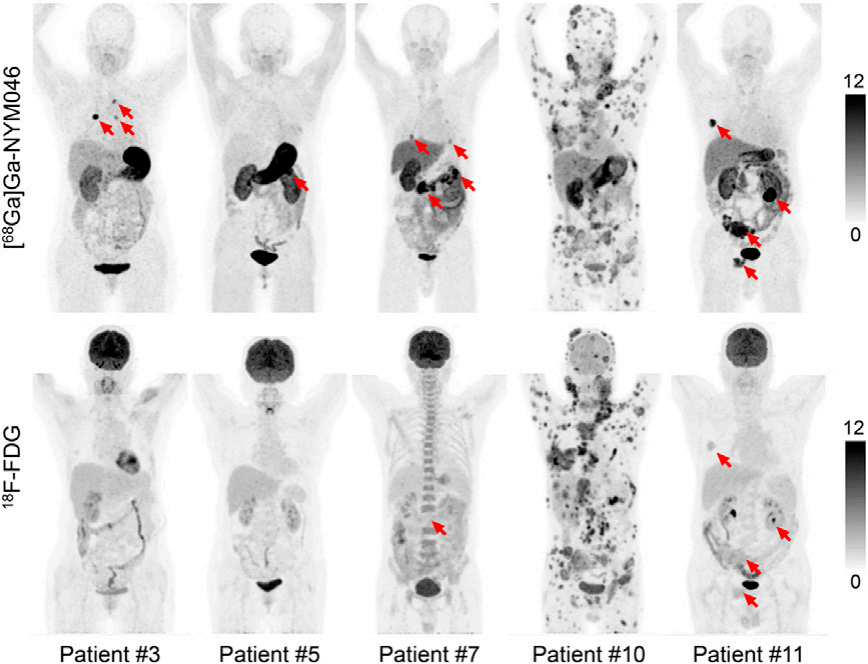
Representative maximum-intensity projections of 5 patients (patients 3, 5, 7, 10, and 11) comparing [^68^Ga]Ga-NYM046 and ^18^F-FDG. Both tracers showed specific retention in bone and lymph node metastases (patients 10 and 11). [^68^Ga]Ga-NYM046 PET/CT outperformed ^18^F-FDG PET/CT in detecting primary tumors, lung metastases, and pleural metastases (patients 3, 5, 7, and 11).
